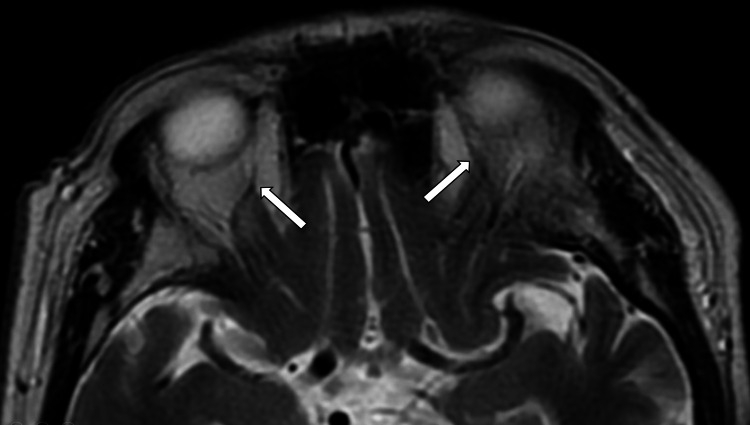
Axial T2-weighted MRI orbit post-treatment. Post-treatment MRI orbit image showed the left and right superior ophthalmic veins (white arrows) return to the normal caliber.
